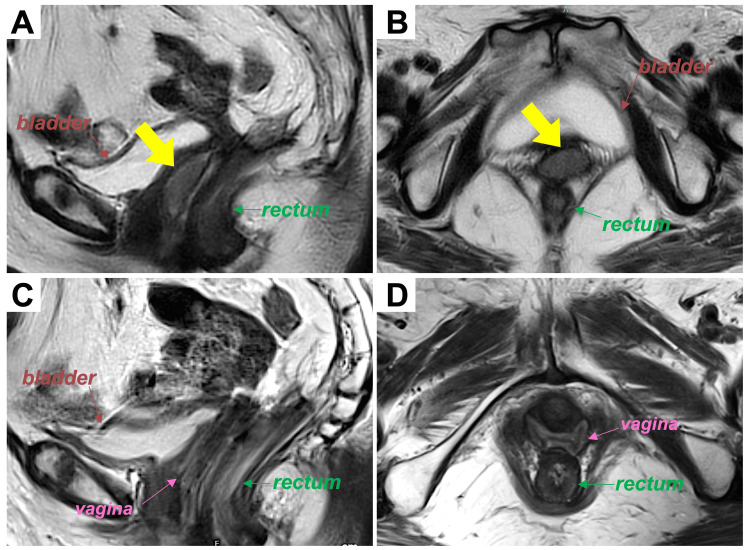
T2-weighted magnetic resonance images of the metastatic vaginal tumor before or six months after radiotherapy (A) Sagittal plane before radiotherapy. (B) Axial plane before radiotherapy. (C) Sagittal plane six months after radiotherapy. (D) Axial plane six months after radiotherapy. Yellow arrows indicate the tumor.
